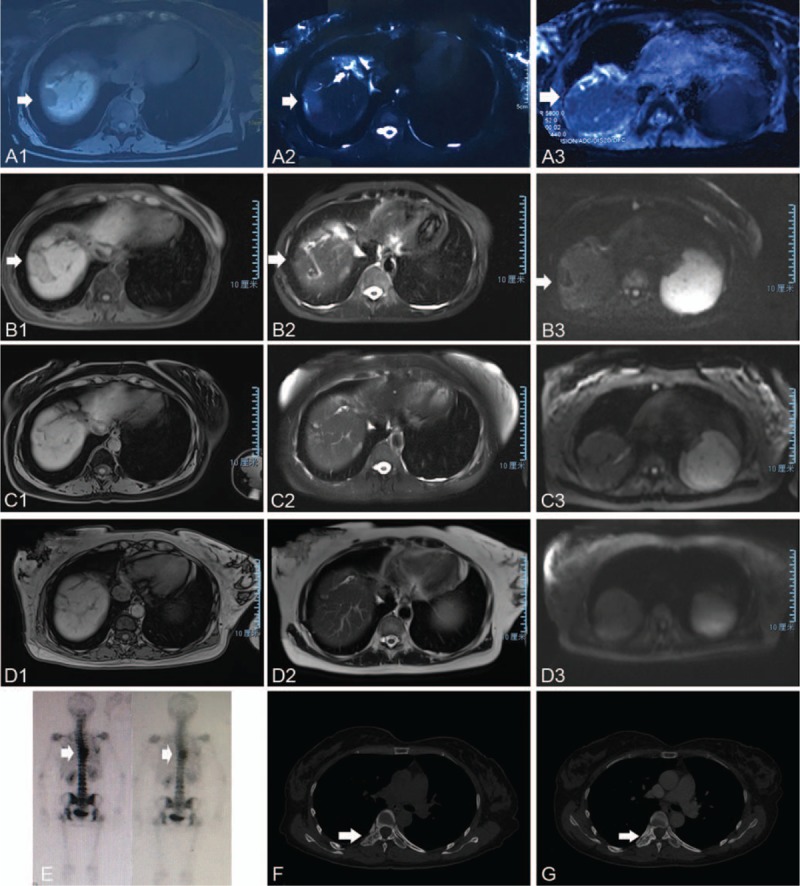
Postoperative radiological examination of the reported case. From (A) to (D), abdominal MRI scanning showed the lesions in the liver was becoming smaller and lessen at time points March 2017, June 2017, May 2018 and January 2019. Figures 3A1, 3B1, 3C1, 3D1 were the T1-weighted magnetic resonance (MR) image; Figures 3A2, 3B2, 3C2, 3D2 were the T2-weighted MR image; Figures 3A3 3B3 3C3 3D3 were the MR with diffusion-weighted images. The white arrow heads direct the liver lesion. (E, F) Emission Computed Tomography (ECT) scanning and bone window of chest routine scan showed metastases in the right thoracic vertebral pedicle at April 2017. (G) T-spine Routine Scan showed the metastases in the right thoracic vertebral pedicle were stable at January 2018. The white arrow heads direct the metastases.
